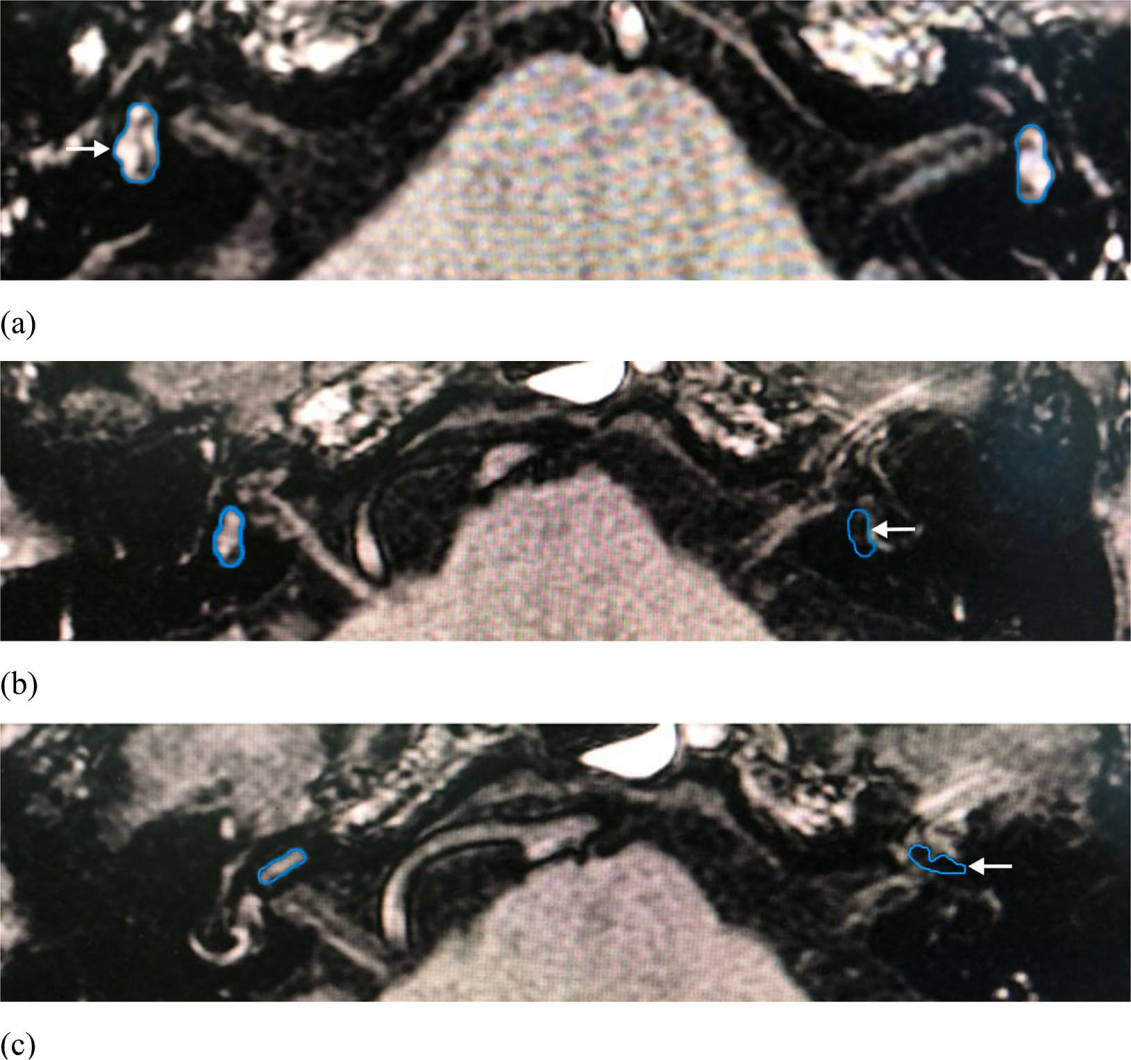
Three-dimensional fluid attenuated inversion recovery (3D-FLAIR) images of patients who diagnosed with definite MD. (a) The region of interest (ROI) placement for the mild endolymphatic hydrops (grade 1) in right-sided vestibule (white arrow). (b) The ROI placement for the obvious endolymphatic hydrops (grade 2) in left-sided vestibule (white arrow). (c) The ROI placement for the significant endolymphatic hydrops (grade 2) in left-sided cochlea (white arrow).
